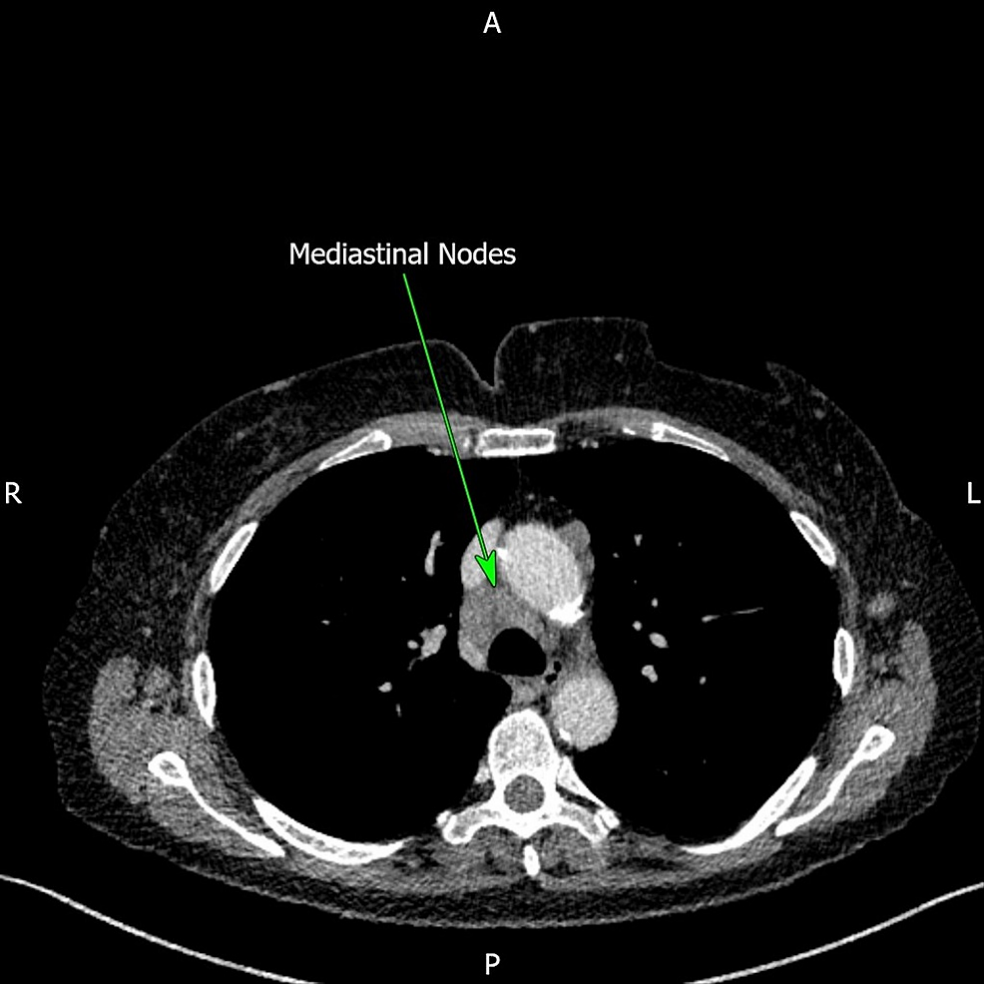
Computed tomography (CT) of the thorax displaying enlarged mediastinal lymph nodes (green arrow).
